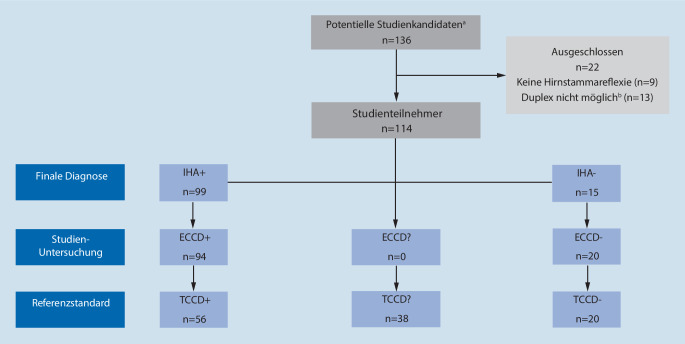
Neun Patienten wurden aufgrund einer inkompletten Hirnstammareflexie ausgeschlossen, bei weiteren 13 Patienten war eine Sonographie nicht erlaubt (keine Richtlinienkonformität gemäß 4. Fortschreibung) oder nicht möglich. Dies war auf große Knochendefekte (*n* = 8) oder ein niedriges Herzzeitvolumen bei Patienten mit vaECMO (*n* = 5) zurückzuführen.
